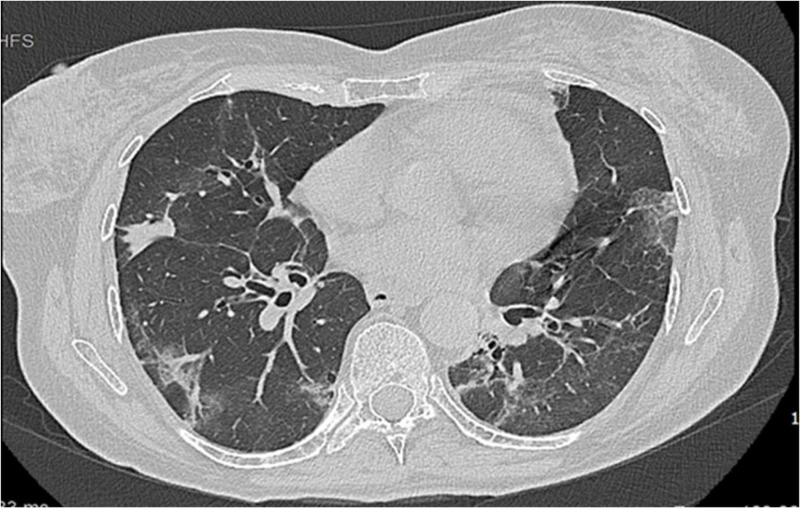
Chest CT scan depicting bilateral SARS-CoV-2 interstitial pneumonia.
